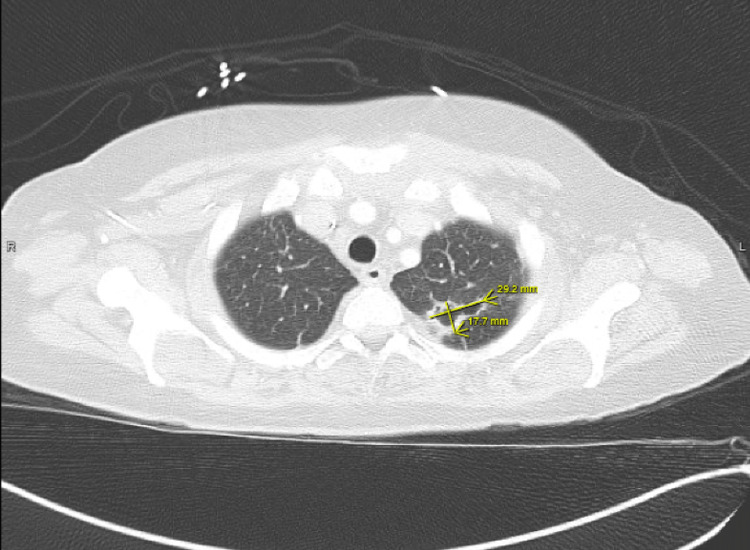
CT scan of the chest (axial slice, lung window) showing septic embolus to left lung field.
